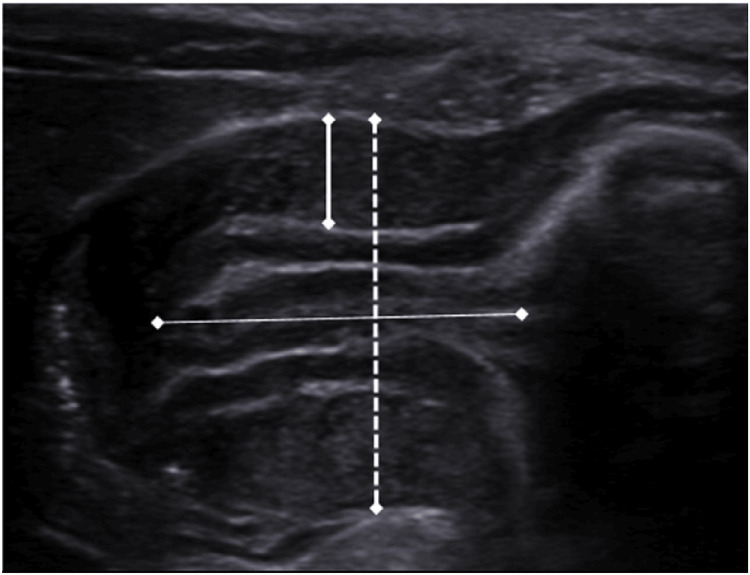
USG abdomen (Case 1) Thickened pylorus measuring 4.5 mm and a symmetrically thickened and edematous wall of length 15 mm, with luminal narrowing. USG, ultrasound
